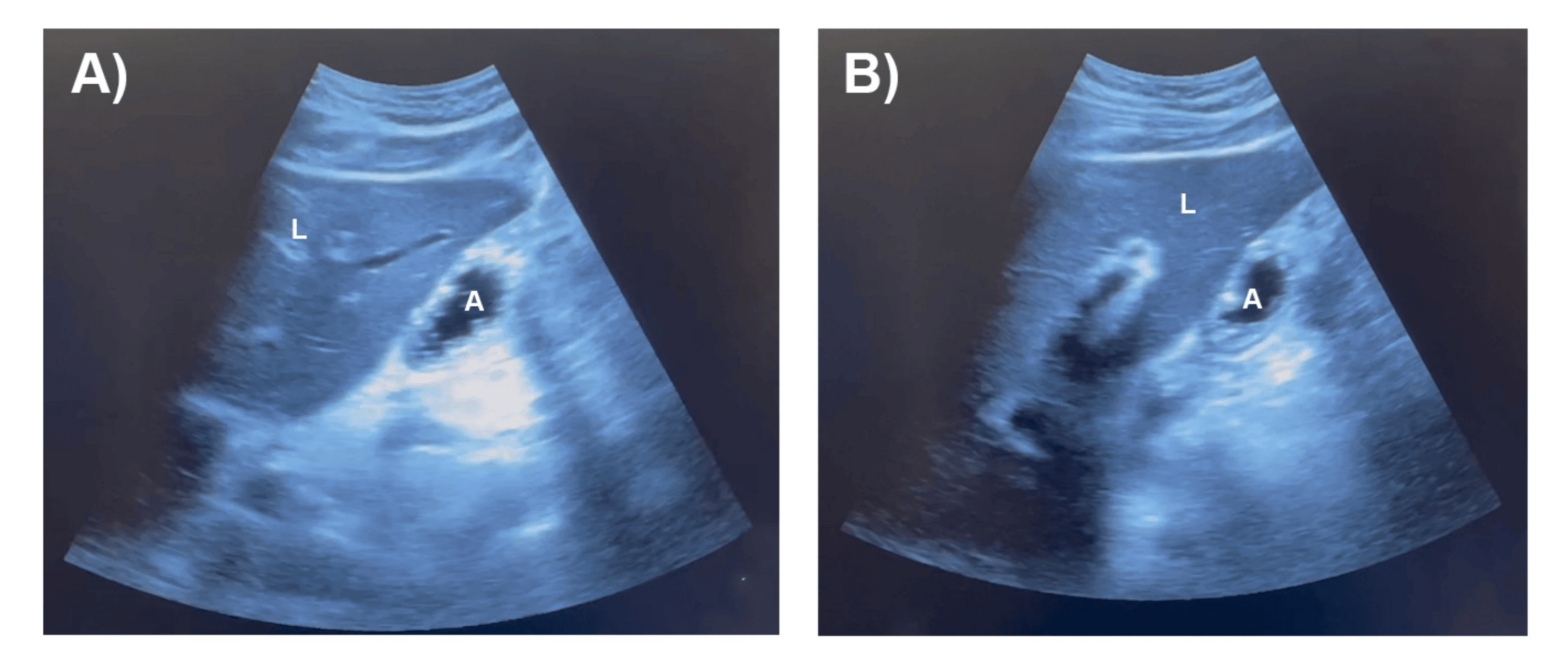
Gastric point-of-care ultrasound demonstrating clear liquids in the antrum in the right lateral decubitus position Pertinent structures were identified, such as the liver (L) and gastric antrum (A). Clear liquids appear homogenous and hypoechoic in the antrum (A, B). After identification of clear liquids, the cross-sectional area is measured to aid in the calculation of the estimated gastric volume. Both images (A and B) were taken from the same patient.
